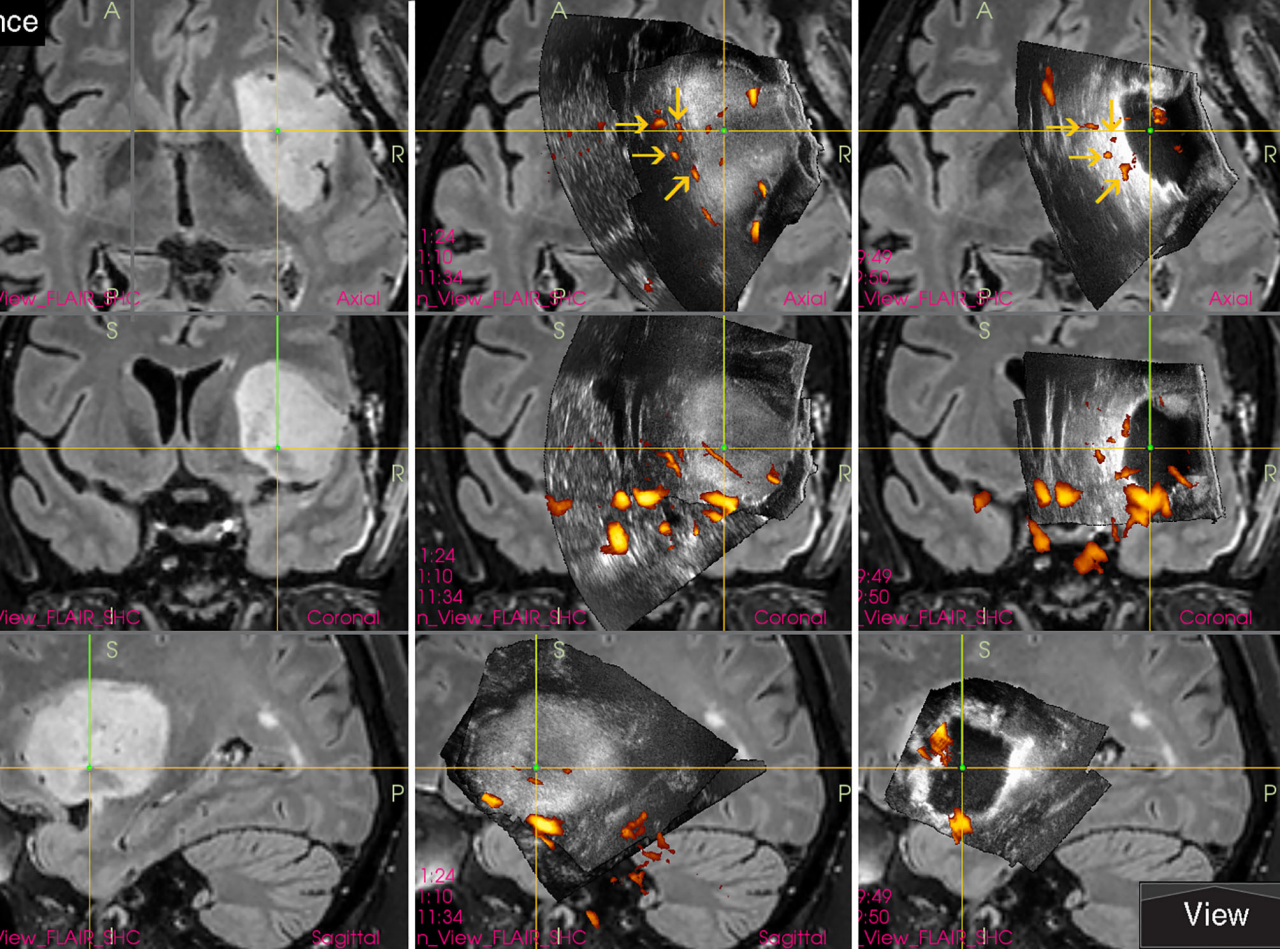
Right-sided insular grade II astrocytoma (Left column) preoperative navigation 3D FLAIR MRI sequence (Middle column) pre-resectional 3D IOUS image fused with navigation FLAIR MRI sequence. Yellow arrows = lenticulostriate arteries visualized by 3D IOUS power-Doppler mode (Right column) 3D IOUS image acquired shortly before the end of resection. Note the close proximity of resection cavity bottom to the lenticulostriate arteries (arrows), intraoperative visualization of perforating arteries helped to prevent iatrogenic injury to them. 3D, three-dimensional; FLAIR, fluid attenuated inversion recovery; MRI, magnetic resonance imaging; IOUS, intraoperative ultrasound.
